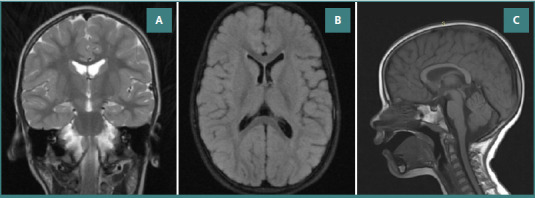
MRI examination. A, Coronal T2 examination with a normal aspect of the cerebral hemisphere, cerebral ventricles, and brainstem. B, Axial FLAIR image without anomalies of white cerebral matter, basal ganglia, and cerebral cortex. C, Sagittal T1 examination without signal or morphological abnormalities in the cerebral hemispheres, brain stem, pericerebral fluid spaces, basal cisterns with morphology within normal limits, and cerebellar tonsils normally located.
